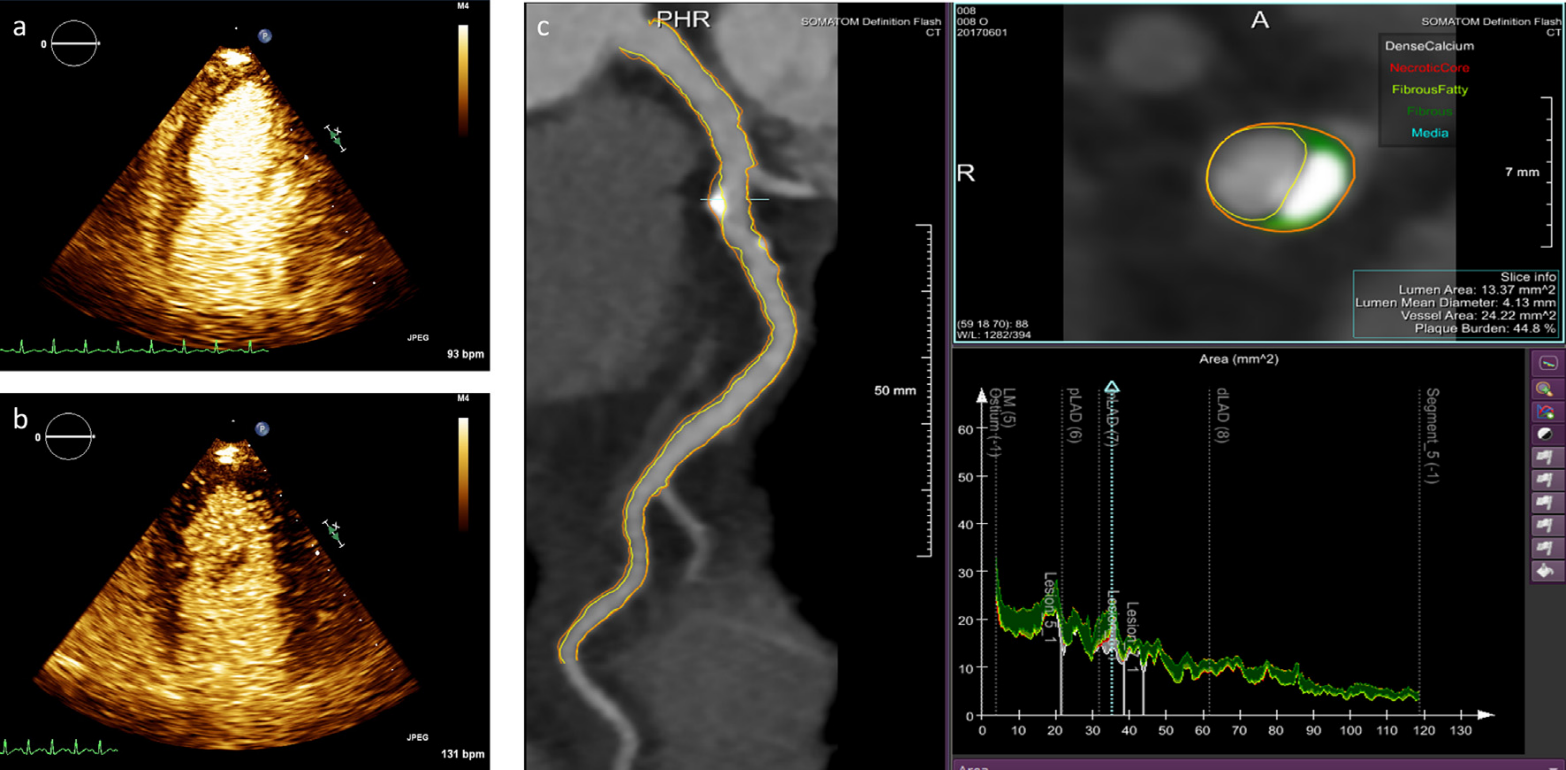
Stress induced myocardial ischemia and quantification of coronary artery plaque burden in a patient with non-obstructive coronary artery disease and chronic coronary syndrome. Myocardial contrast dobutamine stress echocardiography demonstrated normal perfusion at rest (panel a) and myocardial ischemia with delayed myocardial contrast enhancement in the apical part of the left ventricle at peak dobutamine stress (panel b). Quantification of coronary artery plaque burden by coronary computed tomography angiography is shown in panel c.
